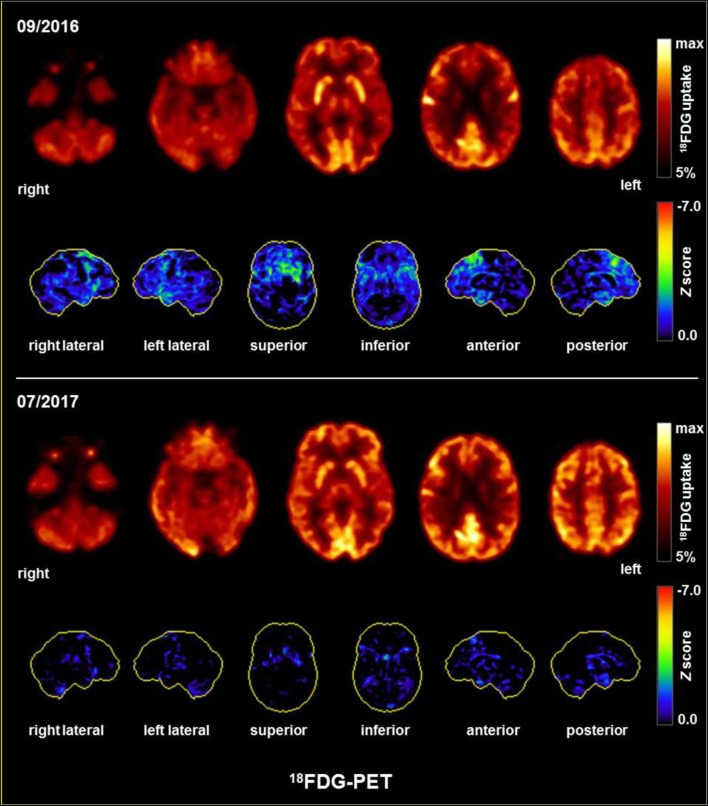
FDG-PET showing mild-to-moderate medial and superior dorsolateral frontal hypometabolism before and normalization after plasmapheresis. The upper and lower row images show the transaxial fluorodeoxyglucose positron emission tomography (FDG-PET) images and the 3D surface projections of the regions with decreased FDG uptake (color-coded *Z*-score and compared with age-matched healthy controls, respectively).
